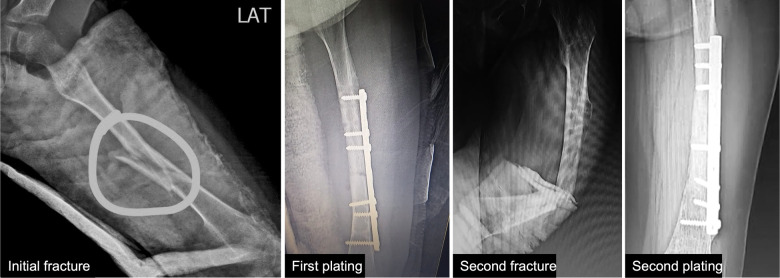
Patient’s x-rays. First x-ray shows the initial left femoral shaft fracture. Second x-ray shows the first plating fixation. Third x-ray shows the fracture after a slight twist of the leg. Fourth x-ray shows the second plating fixation.
